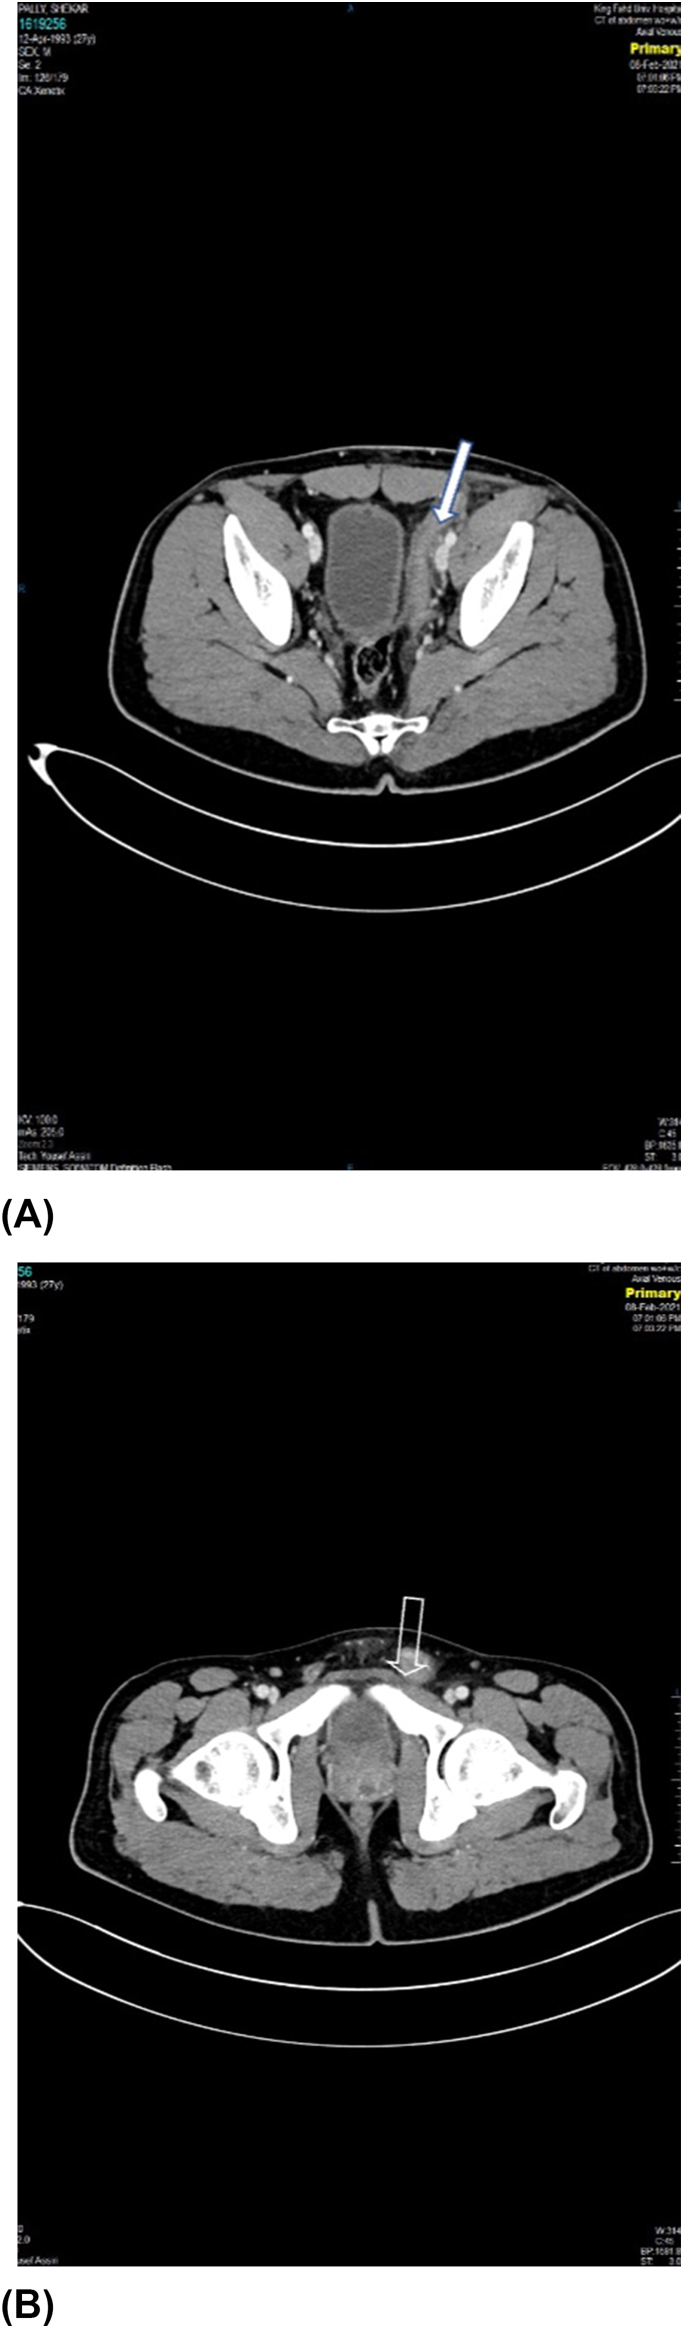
(A , B) Contrast enhanced CT scan axial images showing significantly thickened and swollen left spermatic cord (empty arrow)and vas deference (solid arrow) with surrounding inflammatory fat strandings. A small rim enhancing collection (arrow head) is seen in the most inferior aspect of the left seminal vesicle/left ejaculatory duct measuring 1.5*2.8 mm in maximum dimension.
